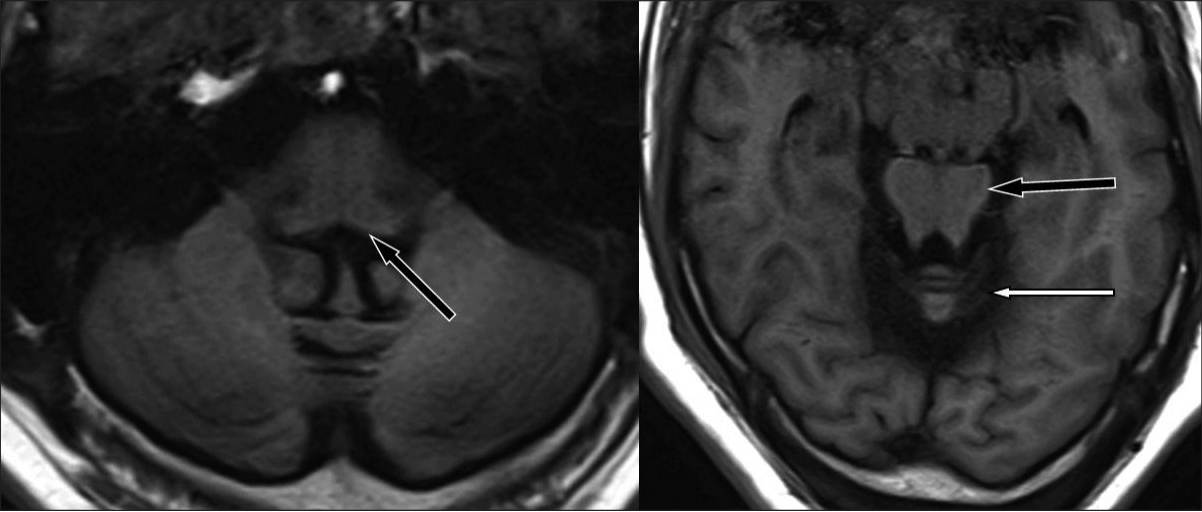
Axial T1WI MR images showing dark outline of the dural surfaces, cerebellum (white arrow) and brainstem (black arrow).
